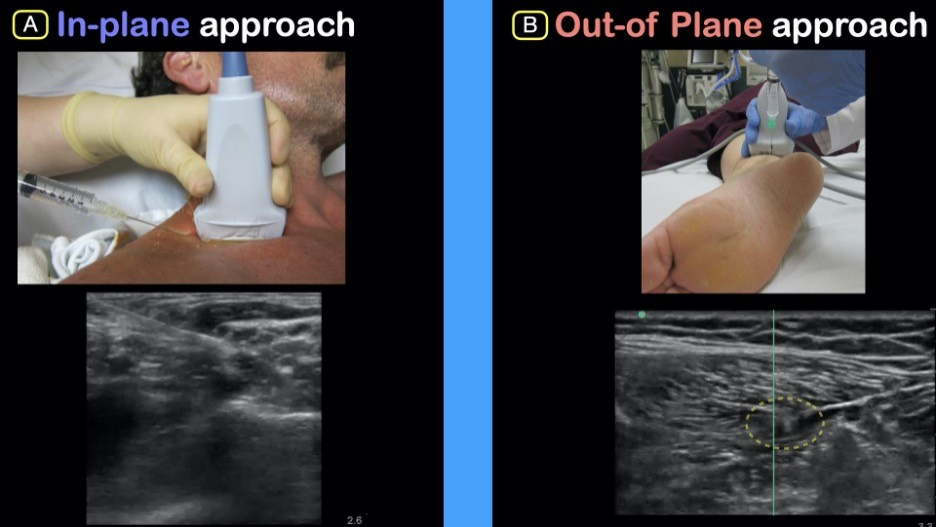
Peripheral Tibial Nerve Block: In-plane approach of needle with visualization of anechoic fluid surrounding nerve on ultrasound.
